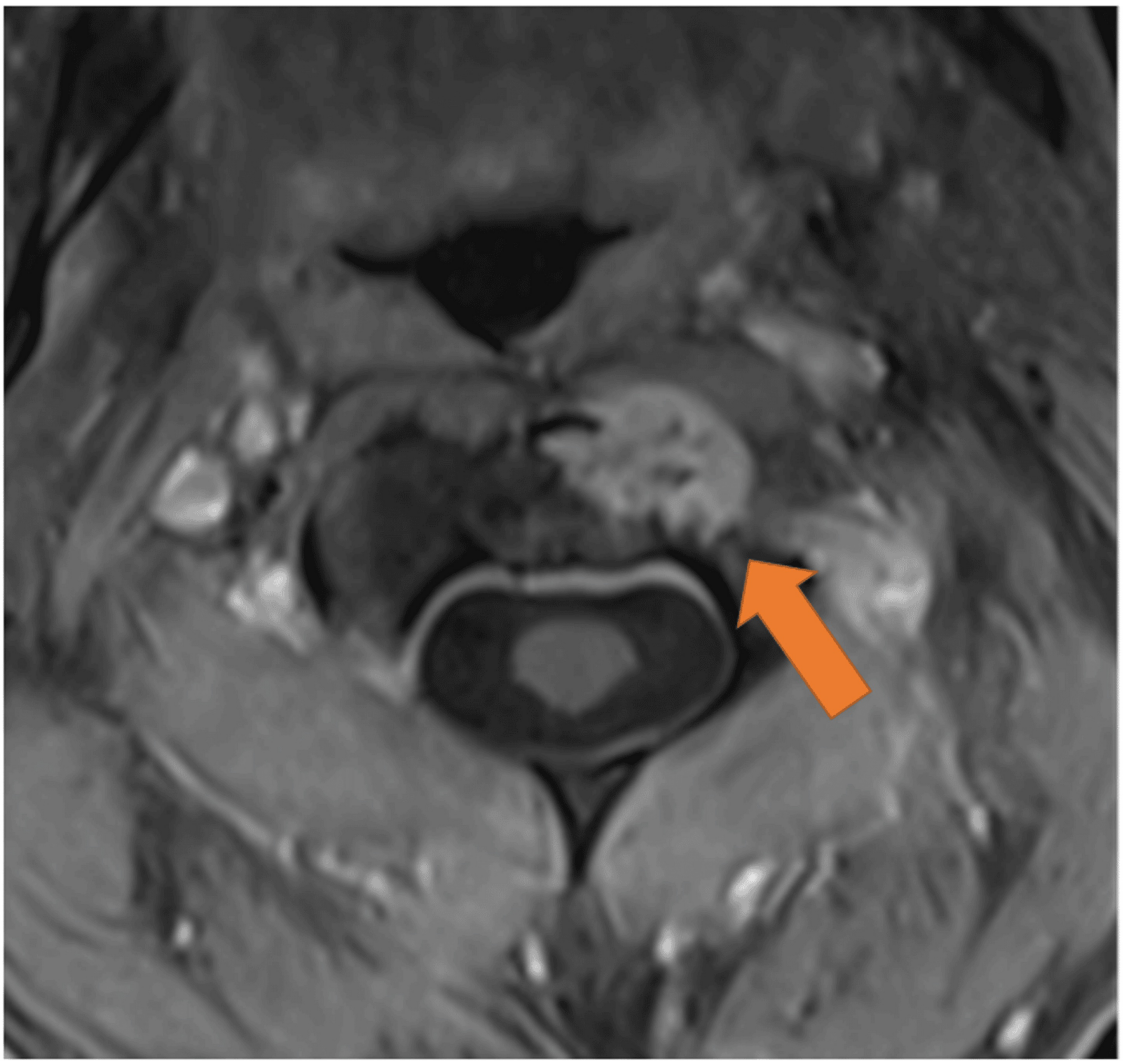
Post-contrast fat-saturated T1-weighted axial MRI image at the level of the C2 lesion shows anterior extraosseous disease uplifting the left longus colli muscle (arrow).
